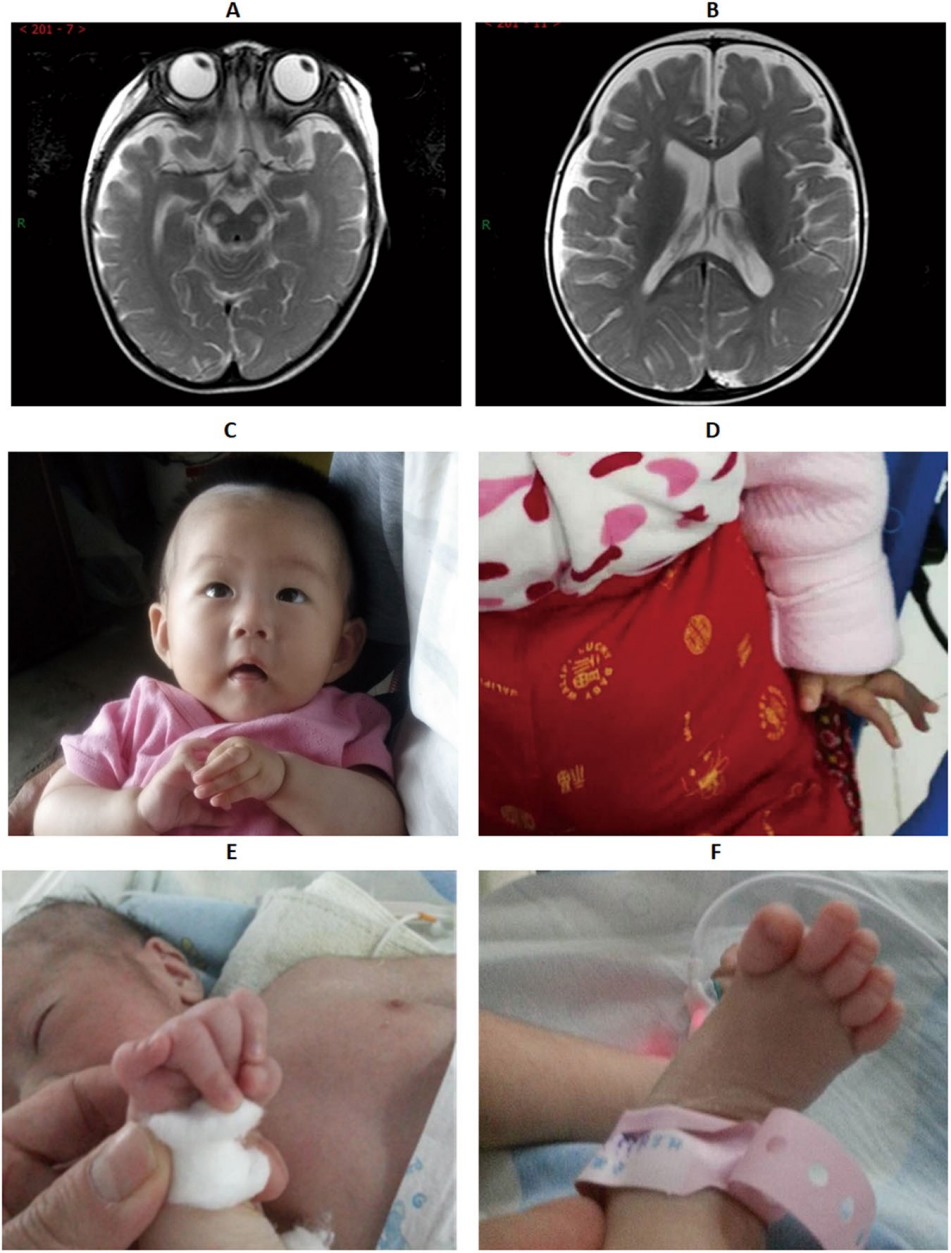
Clinical findings in patients with developmental delay accompanied unexplained dyspnea. For patient 16, long T1 and long T2 signal was revealed by cranial MRI (A) and long T1 signal was noted at sagittal medulla oblongata in T1WI. Patient 24 lapsed into unconsciousness, with opening eyes and contracture of fingers (A,B). Dysmorphic facial features including low set ears and micromandible together with contracture of fingers and toes were noted in patient 31 (C,D).
